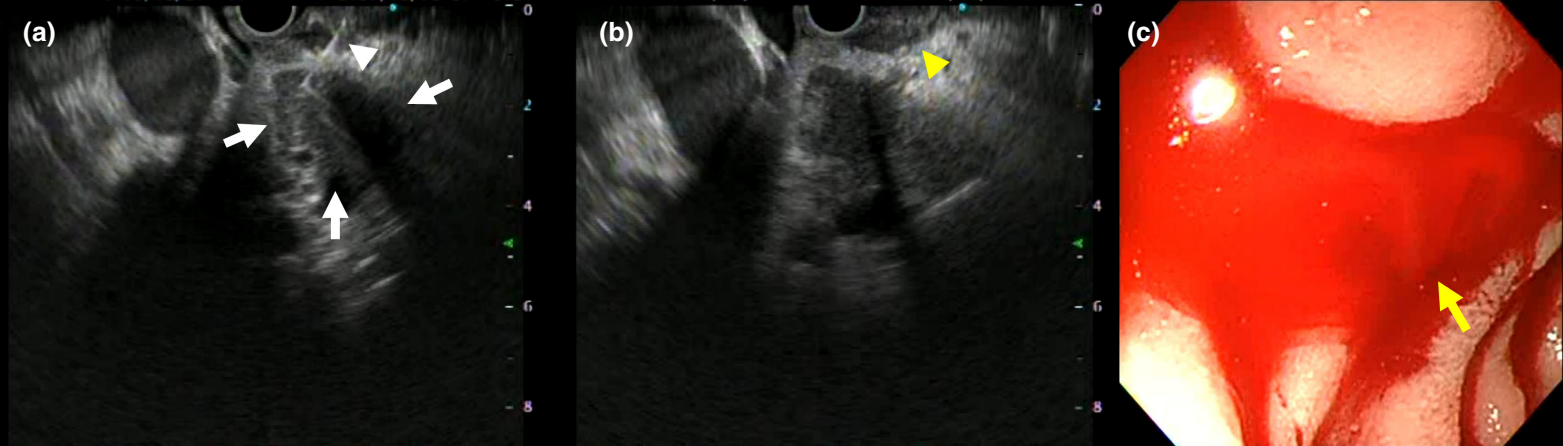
(a) Endoscopic ultrasound‐guided tissue acquisition was performed for a pancreatic tumor (white arrows) from the duodenal bulb using a 25G Franseen needle (white arrowhead). (b) There was no intraperitoneal or intramural bleeding (yellow arrowhead). (c) Spurting hemorrhage occurred from the puncture site (yellow arrow).
